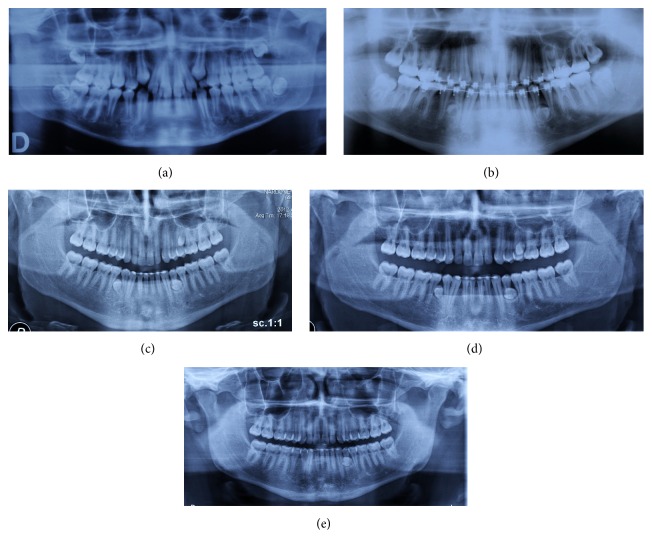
Case 2 radiography: before treatment 2006 (a), before rebonding, ST in regions 3.4–3.5, 4.4–4.5, and 2.5–2.6, 2009 (b), 3 years after debonding 2012 (c), 4 years after debonding 2013 (d), and after the extractions of ST in regions 4.4–4.5 and 2.5–2.6, 2015 (e).
